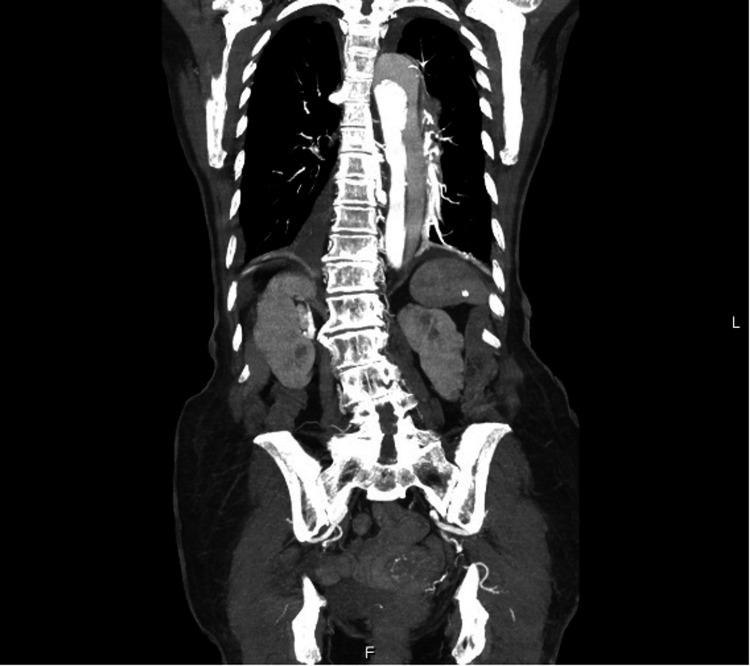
Chest, abdominal, and pelvic CT with a thoracic aortic dissection, DeBakey type I, Stanford type A, extending to the abdominal aorta, the emergence of the celiac trunk in the false lumen and superior mesenteric artery with the emergence in the true lumen
